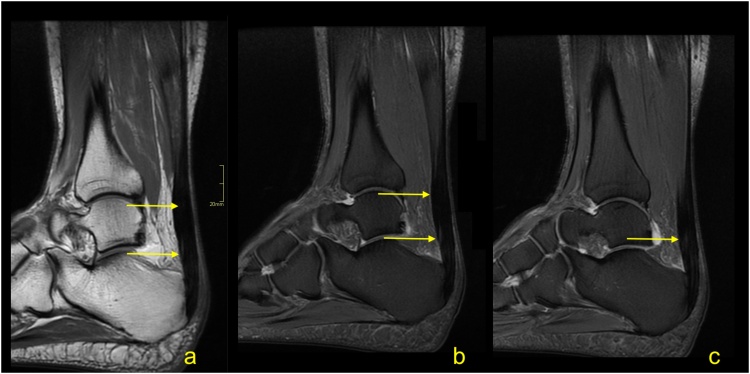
Tendinopathy of the whole Achilles tendon (arrow) in a 62-year-old patient with pain for more than 5 years. The MRI revealed thickening of the whole Achilles tendon (arrow). A- PD-weighted, b and c- PD-weighted with fat suppression.
